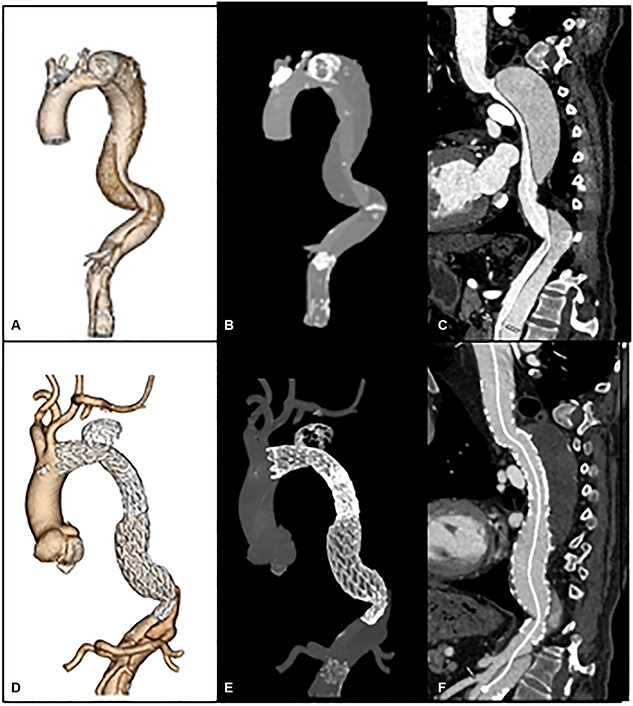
Preoperative computed tomography angiography imaging of a chronic type B dissection with aneurysm.
